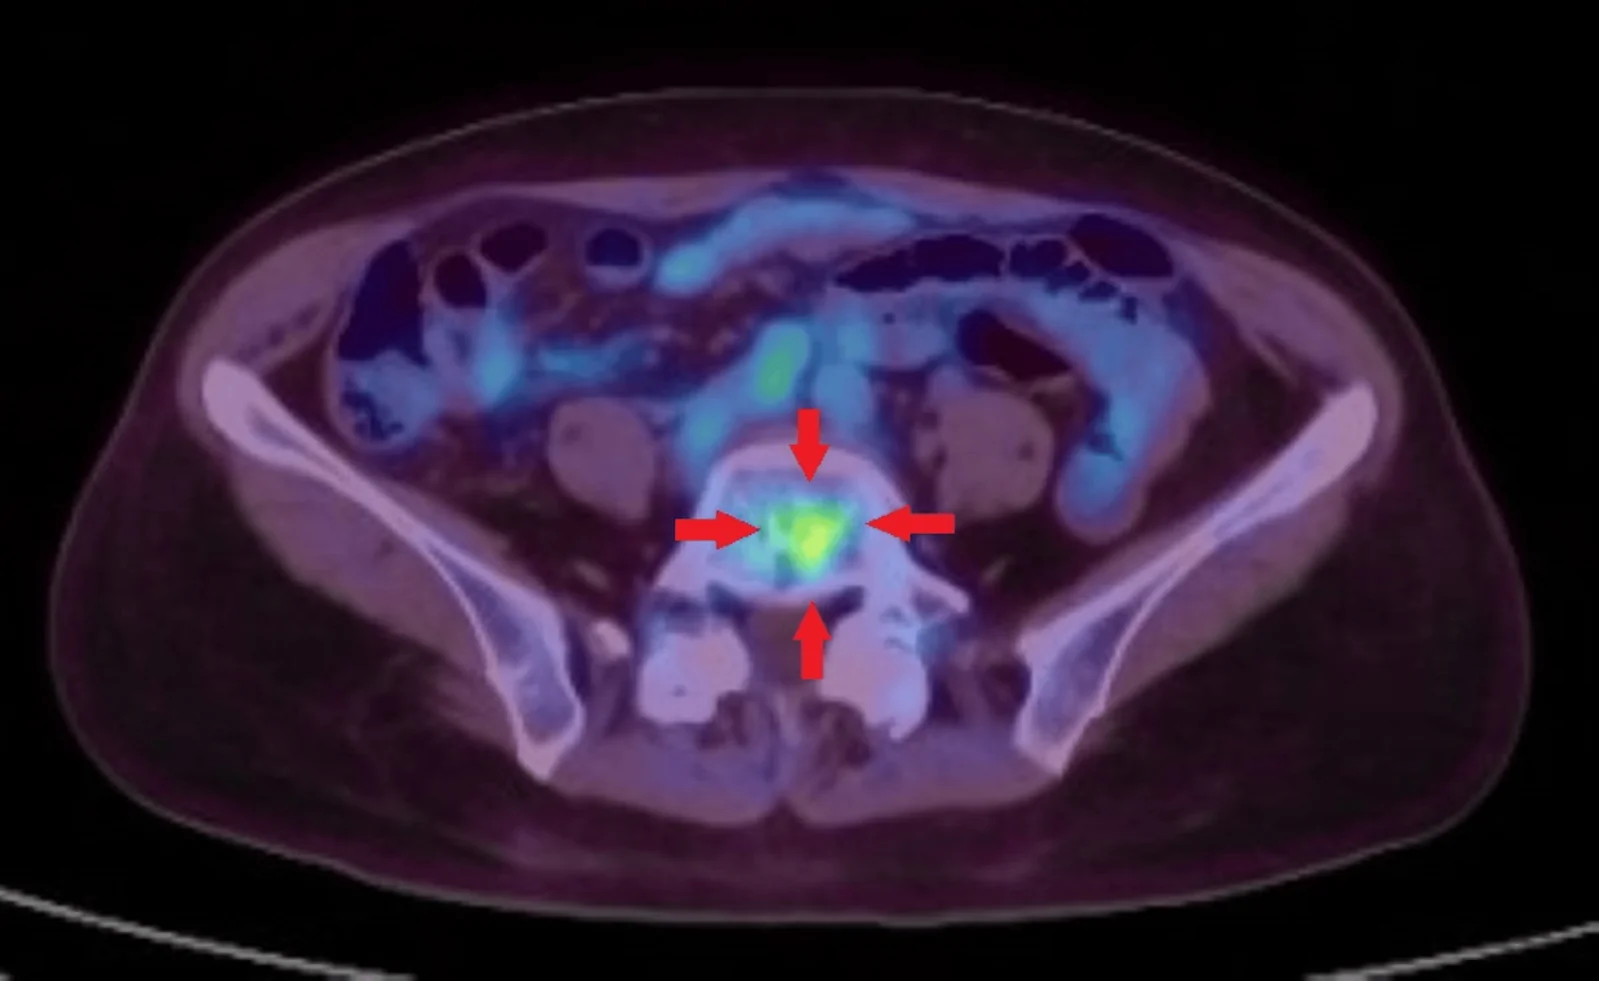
FDG PET-CT findings of the fifth lumbar vertebra FDG PET-CT showing increased FDG uptake in the fifth lumbar vertebra. The findings were suggestive of possible osseous involvement by lymphoma. FDG: fluorodeoxyglucose; PET-CT: positron emission tomography-computed tomography
